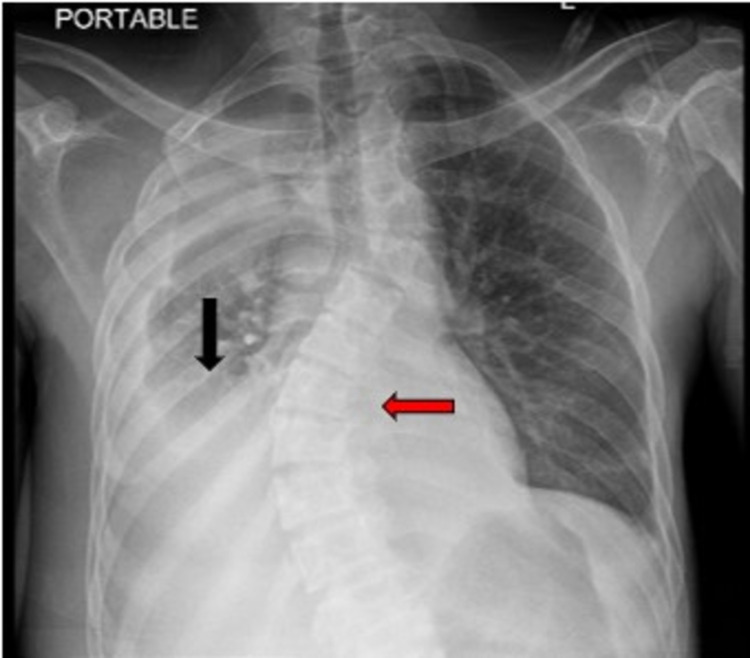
X-ray showing pleural effusions secondary to pneumonia (black arrow), in addition to chronic stable, S-shaped scoliosis (red arrow).
